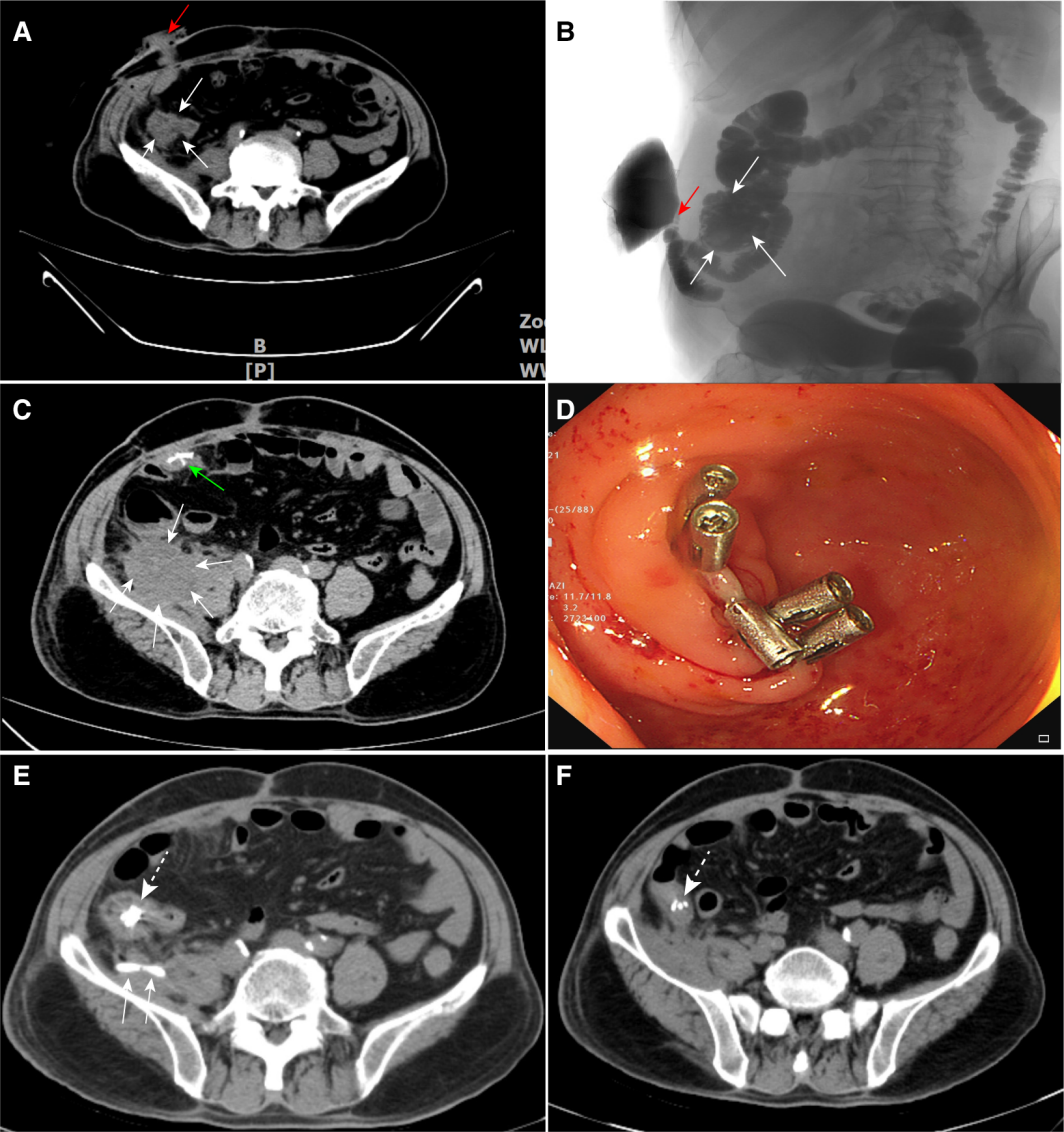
(A,B) Assessment of the baseline condition prior to reversal of ileal stoma: the white arrows indicate the cecum, and the red arrows represent the terminal ileal stoma. (C) The white arrows indicate the encapsulated abscess in the right iliac fossa, while the green arrow shows the titanium staples at the ileal anastomosis site. (D) Five titanium clips were clamped on the appendiceal orifice during colonoscopy. (E) On CT, the white dotted arrow indicates the titanium clips, and the white arrows display the J-catheter within the abdominal cavity. (F) Follow-up abdominal CT shows that the abscess has completely resolved. The white dotted arrow indicates the titanium clips. CT, computed tomography.
